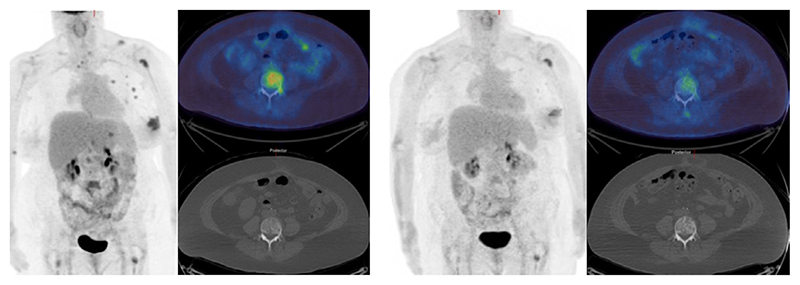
^18^F-FDG PET/CT (maximum intensity projection image - left, axial images through L4 - right) show high uptake in a primary left breast cancer and bone metastases with some underlying sclerosis on the CT component. After 8 weeks of endocrine therapy a partial metabolic response is visible (L4 metastasis SUVmax decreased from 8.4 to 4.4).
